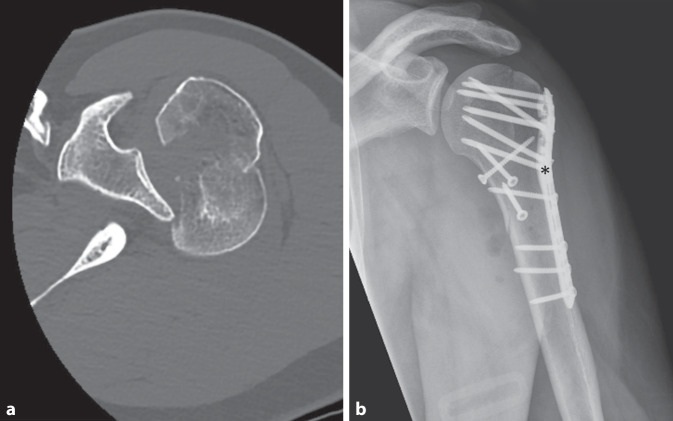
Aufnahmen eines 45-jährigen Patienten nach einem Verkehrsunfall mit posteriorer Luxationsfraktur Typ 5 nach Resch et al. [22]. a Präoperative Schicht der axialen CT-Bildgebung, b postoperative Röntgenbildgebung nach winkelstabiler Platten- und Schraubenosteosynthese. *Asteriskus* Kalkarschraube
